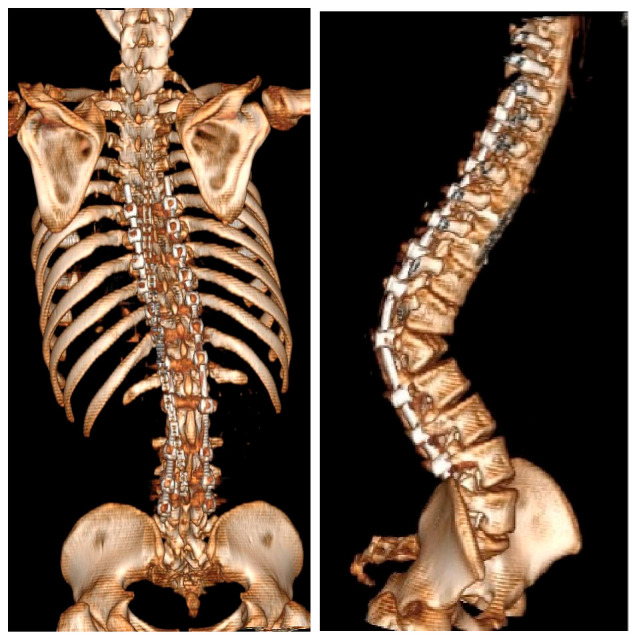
3D-CT after the first stage of planned surgical treatment.
